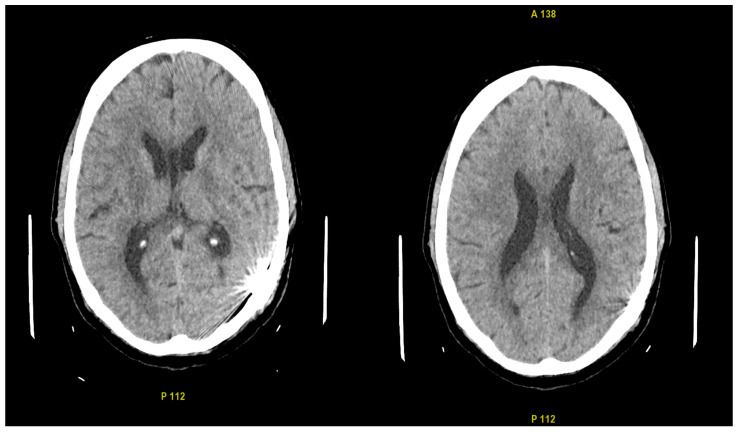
CT Images, Axial View (3 Months Post-Embolization): The images demonstrate normalization of brain morphology, with embolization artifacts visible in the region of the left occipital lobe.
